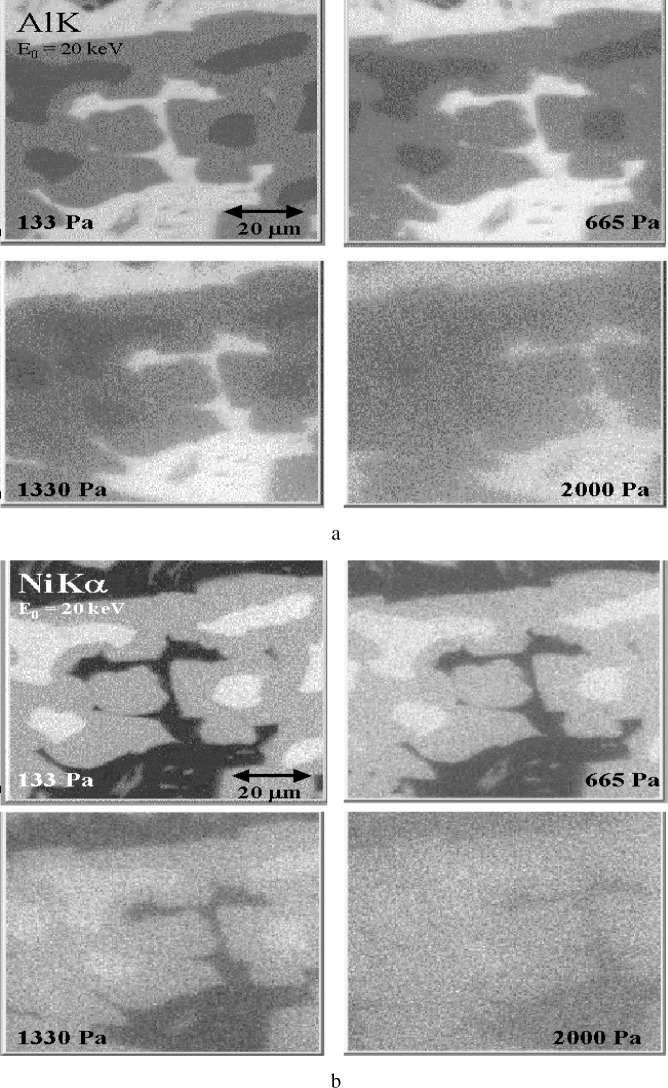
X-ray mapping in the VPSEM/ESEM: Raney nickel, *E*_0_ = 20 keV; all maps at 4 mm gas path; H_2_O at pressures of 133 Pa (1 Torr), 665 Pa (5 Torr), 1330 Pa (10 Torr), 2000 Pa (15 Torr); *E*_0_ = 20 keV; (a) Al x-ray maps; (b) Ni x-ray maps.
